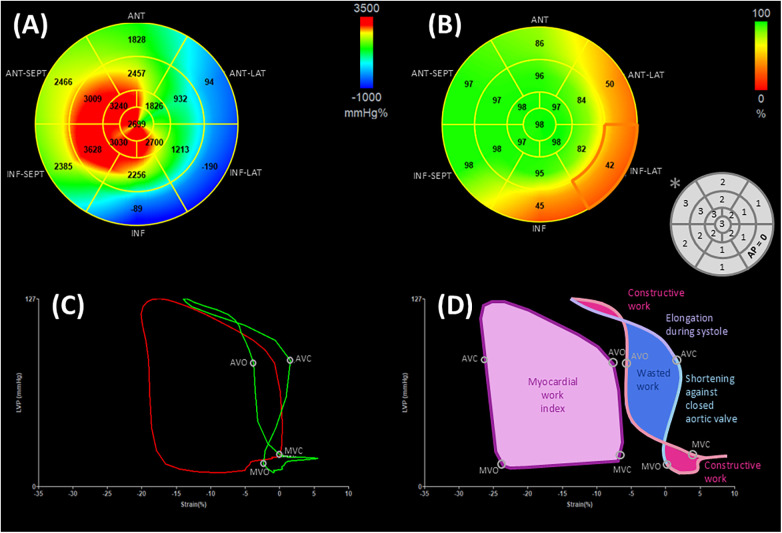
Myocardial work analysis in a patient with WPW syndrome and left inferolateral AP. The bull's eye plot represents LV myocardium divided into individual segments and shows: (A) Segmental values of MWI in mmHg%; (B) Values of MWE in %; (C) Global LV pressure-strain loop (red) and pressure-strain loop representing the segment at ventricular AP insertion (green), where pressure-strain loop area from mitral valve closure to mitral valve opening stands for performed myocardial work, as explained further in the text (see Methods). Major decrease of both MWI and MWE along with a distorted pressure-strain loop is evident in segments adjacent to AP. (D) Graphical presentation of the methodology of MWI, MCW and MWW calculations (30). *Annotation of segmental distance from ventricular accessory pathway AP insertion. ANT, anterior; ANT-LAT, anterolateral; ANT-SEPT, anteroseptal; AP, accessory pathway; AVC, aortic valve closure; AVO, aortic valve opening; INF, inferior; INF-LAT, inferolateral; INF-SEPT, inferoseptal; LV, left ventricular; LVP, left ventricular pressure; MVC, mitral valve closure; MVO, mitral valve opening; MWE, myocardial work efficiency; MWI, myocardial work index; WPW, Wolff-Parkinson-White. Figure 2 consists of screenshots from the EchoPAC platform (see the Echocardiographic examination, Methods section) and graphics created by the first author.
